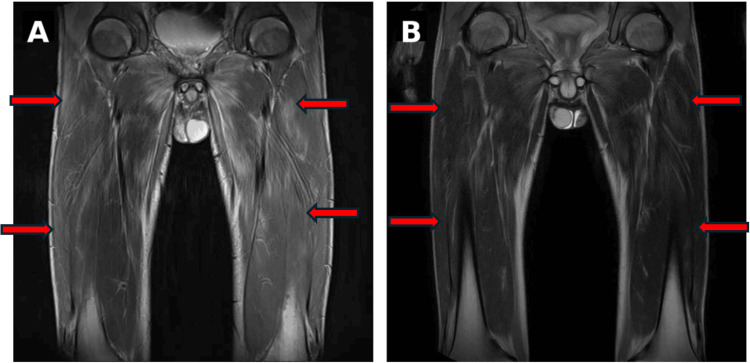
Thigh MRI. (A) Hospital day 1: Diffuse, symmetric T2 hyperintensity in the gluteal and thigh muscles (arrows). (B) Hospital day 28: Decreased T2 signal intensity in the corresponding muscles (arrows), consistent with improvement of myositis.
